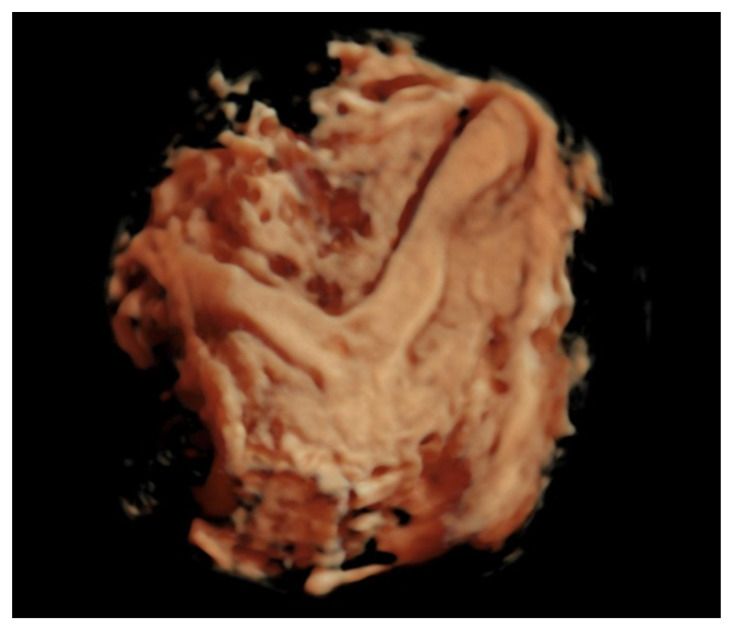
Post-partum ultrasound aspect of the uterus that confirms the suspicion of a bicorn uterus.
